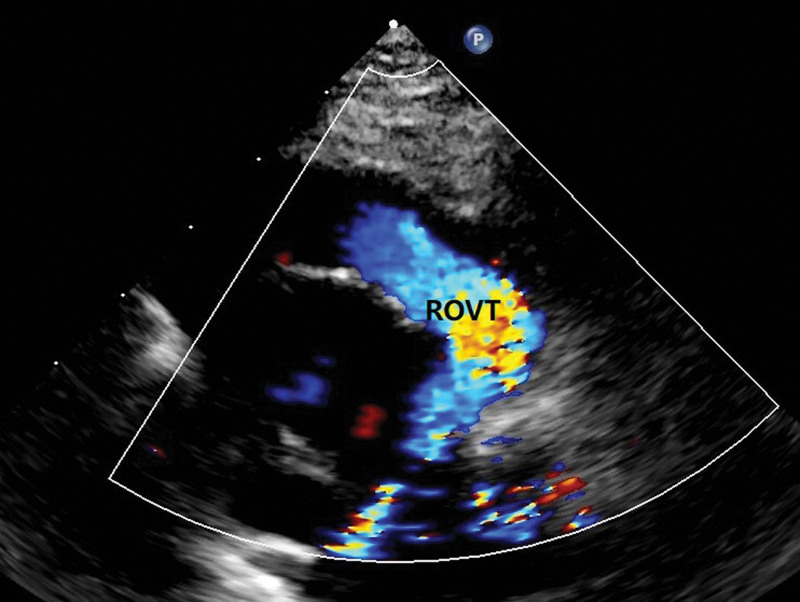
The transthoracic echocardiography (TTE) follows up at 3 months.
